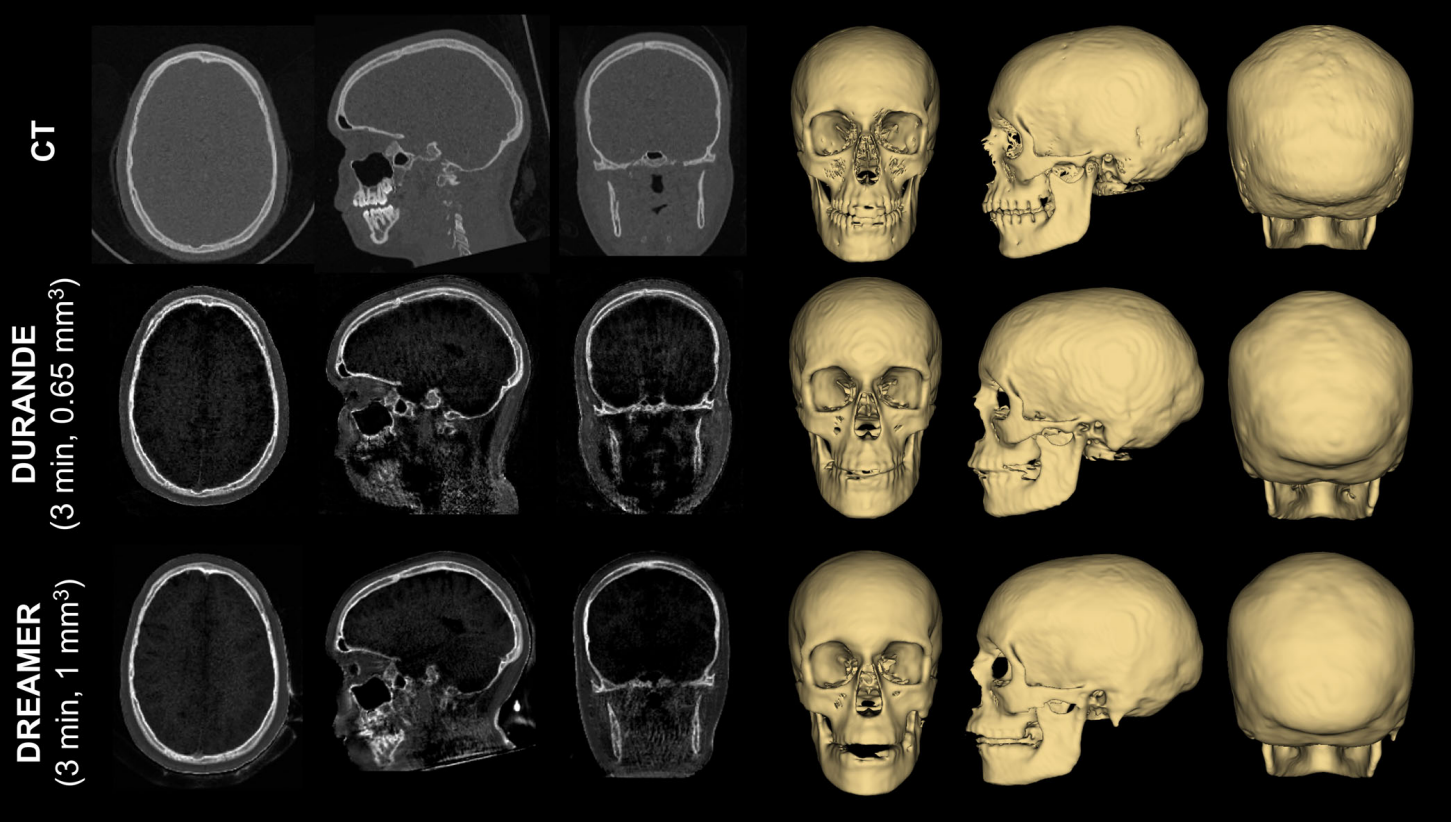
DURANDE (dual‐RF, dual‐echo UTE) and DREAMER bone‐selective images validated with CT for a 17‐year‐old female pediatric patient with fibrous dysplasia and no history of prior cranial vault surgery. DURANDE and DREAMER share the same contrast mechanism for bone‐selective imaging, and hence, full 3D skull renderings from the MR bone‐selective images can be generated from the same U‐Net model previously trained only on DURANDE images.
